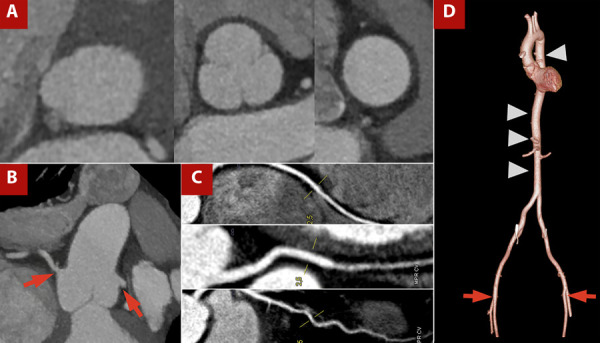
TAVI protocol CT angiography with a one-step approach, including coronary CT angiography. A) Orthogonal measurements at the aortic valve plane, sinuses of Valsalva, and sinotubular junction. B) Assessment of coronary ostial height and origin (red arrow). C) Curved multiplanar reconstruction of coronary arteries showing atherosclerotic disease. D) 3D reconstruction of the thoracoabdominal aorta (arrowhead) and femoral access routes (red arrow).
